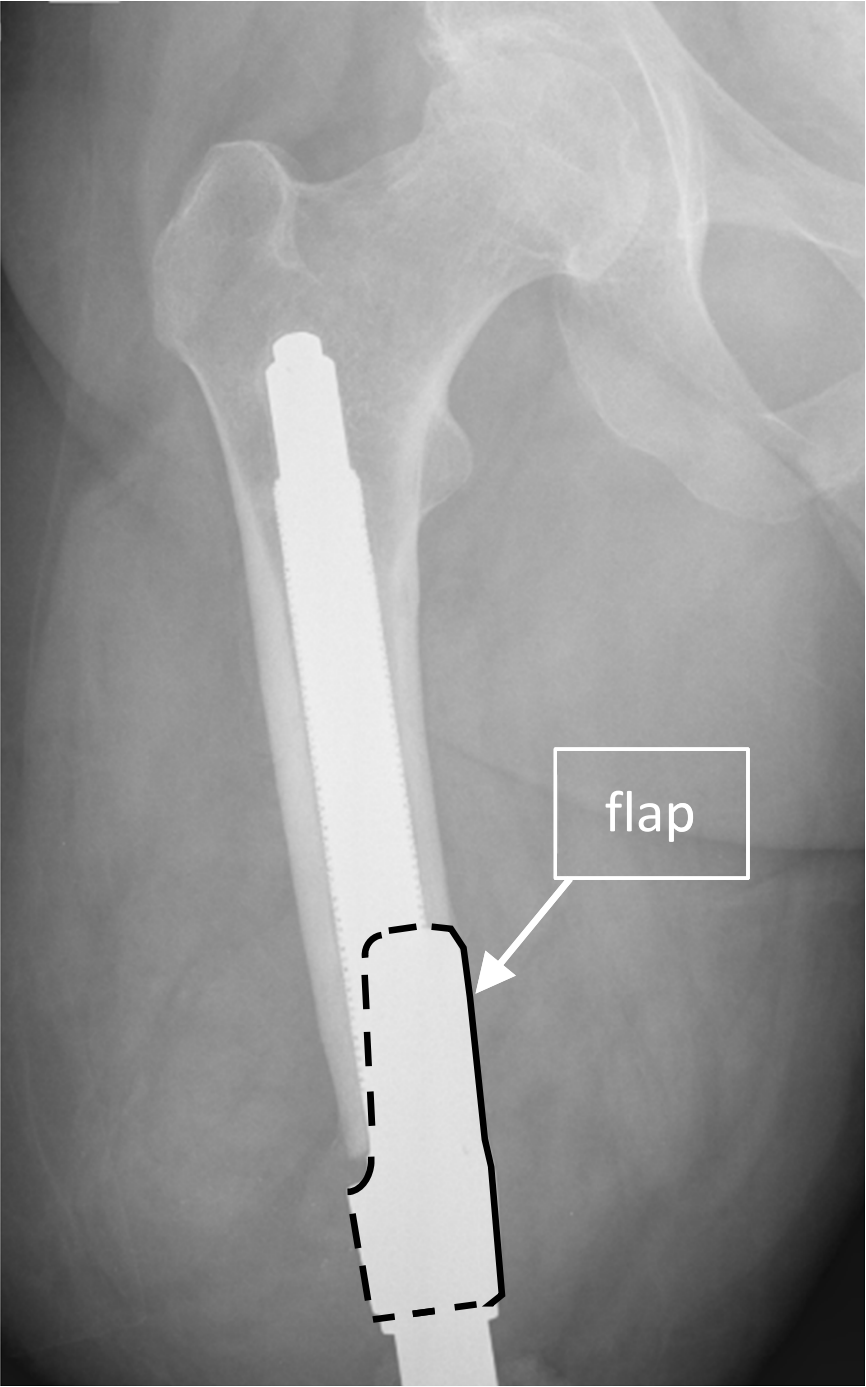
Postoperative x-ray after implantation of an Endo-Fix-Stem. The flap, which was used for the older stems is framed with a dotted and continuous line in black. It was not possible to measure the corticalis thickness in this area
